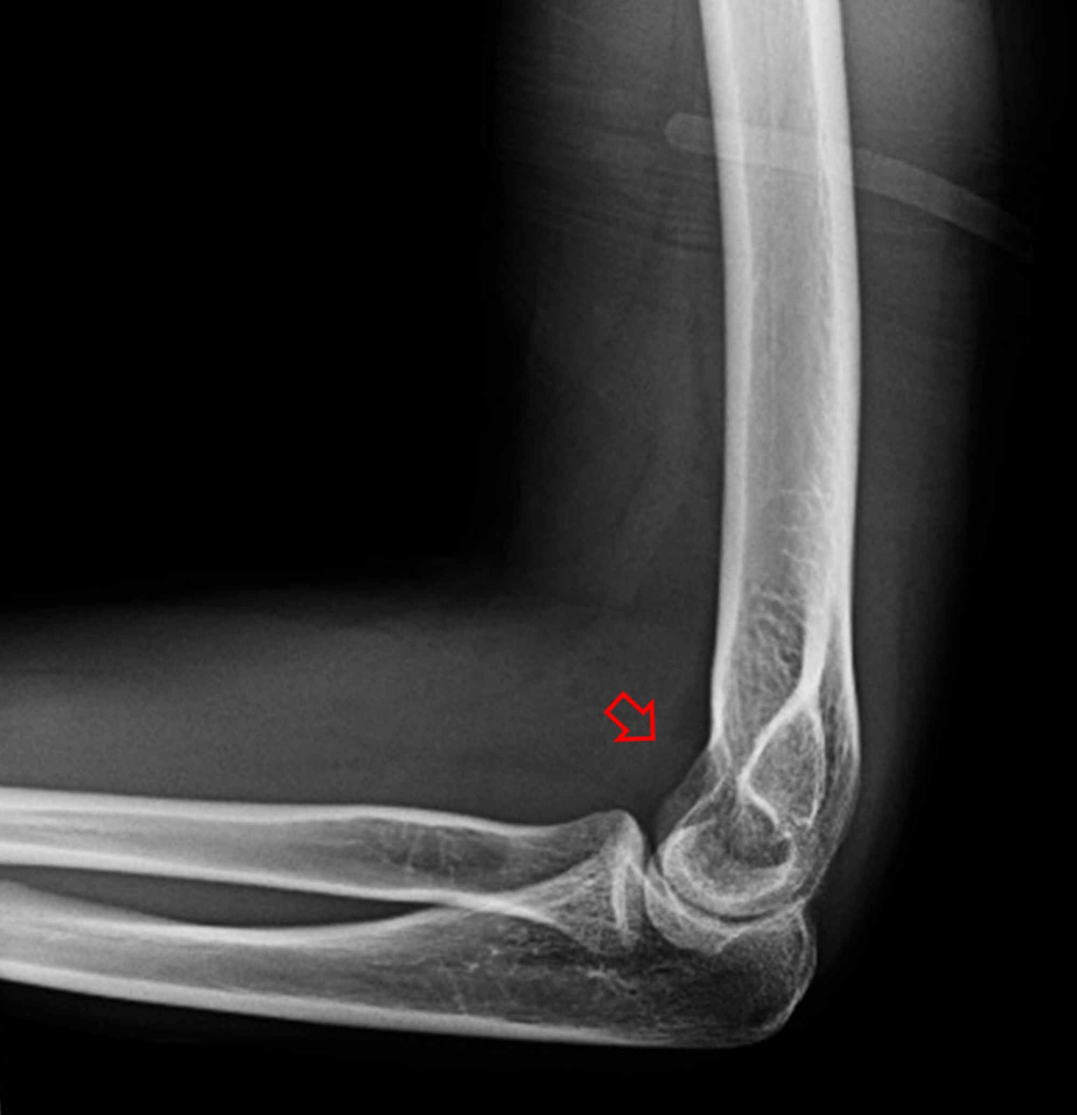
Post-reduction lateral X-ray of the elbow showing reduction of the radial head and elbow joint. Suspected hemarthrosis is indicated by the arrow.
